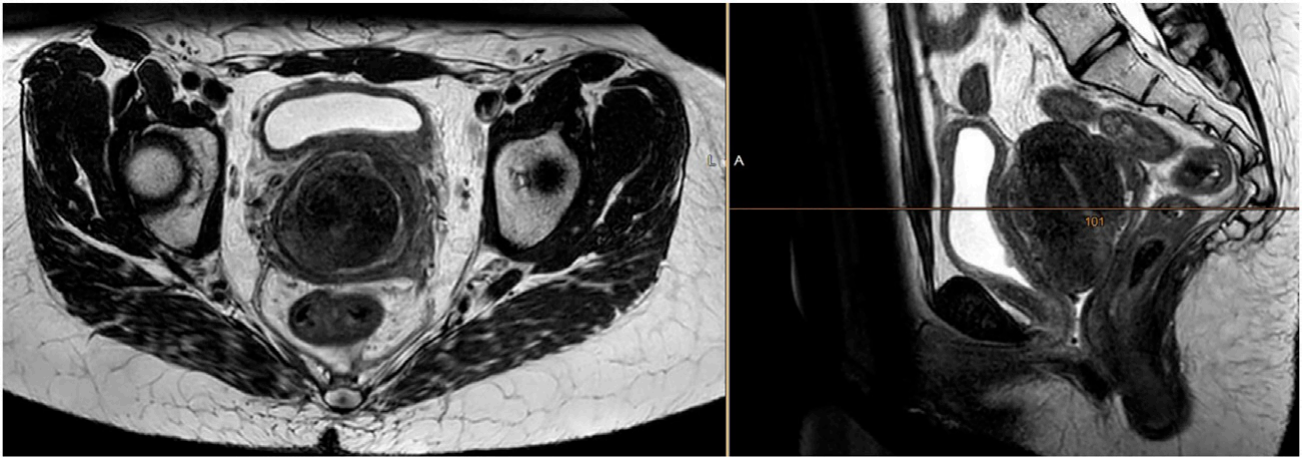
MRI confirming a partial tumor response 1 month after completion of radiotherapy at a dose of 59.4 Gy in 33 fractions. The treatment response was obvious, however incomplete.
